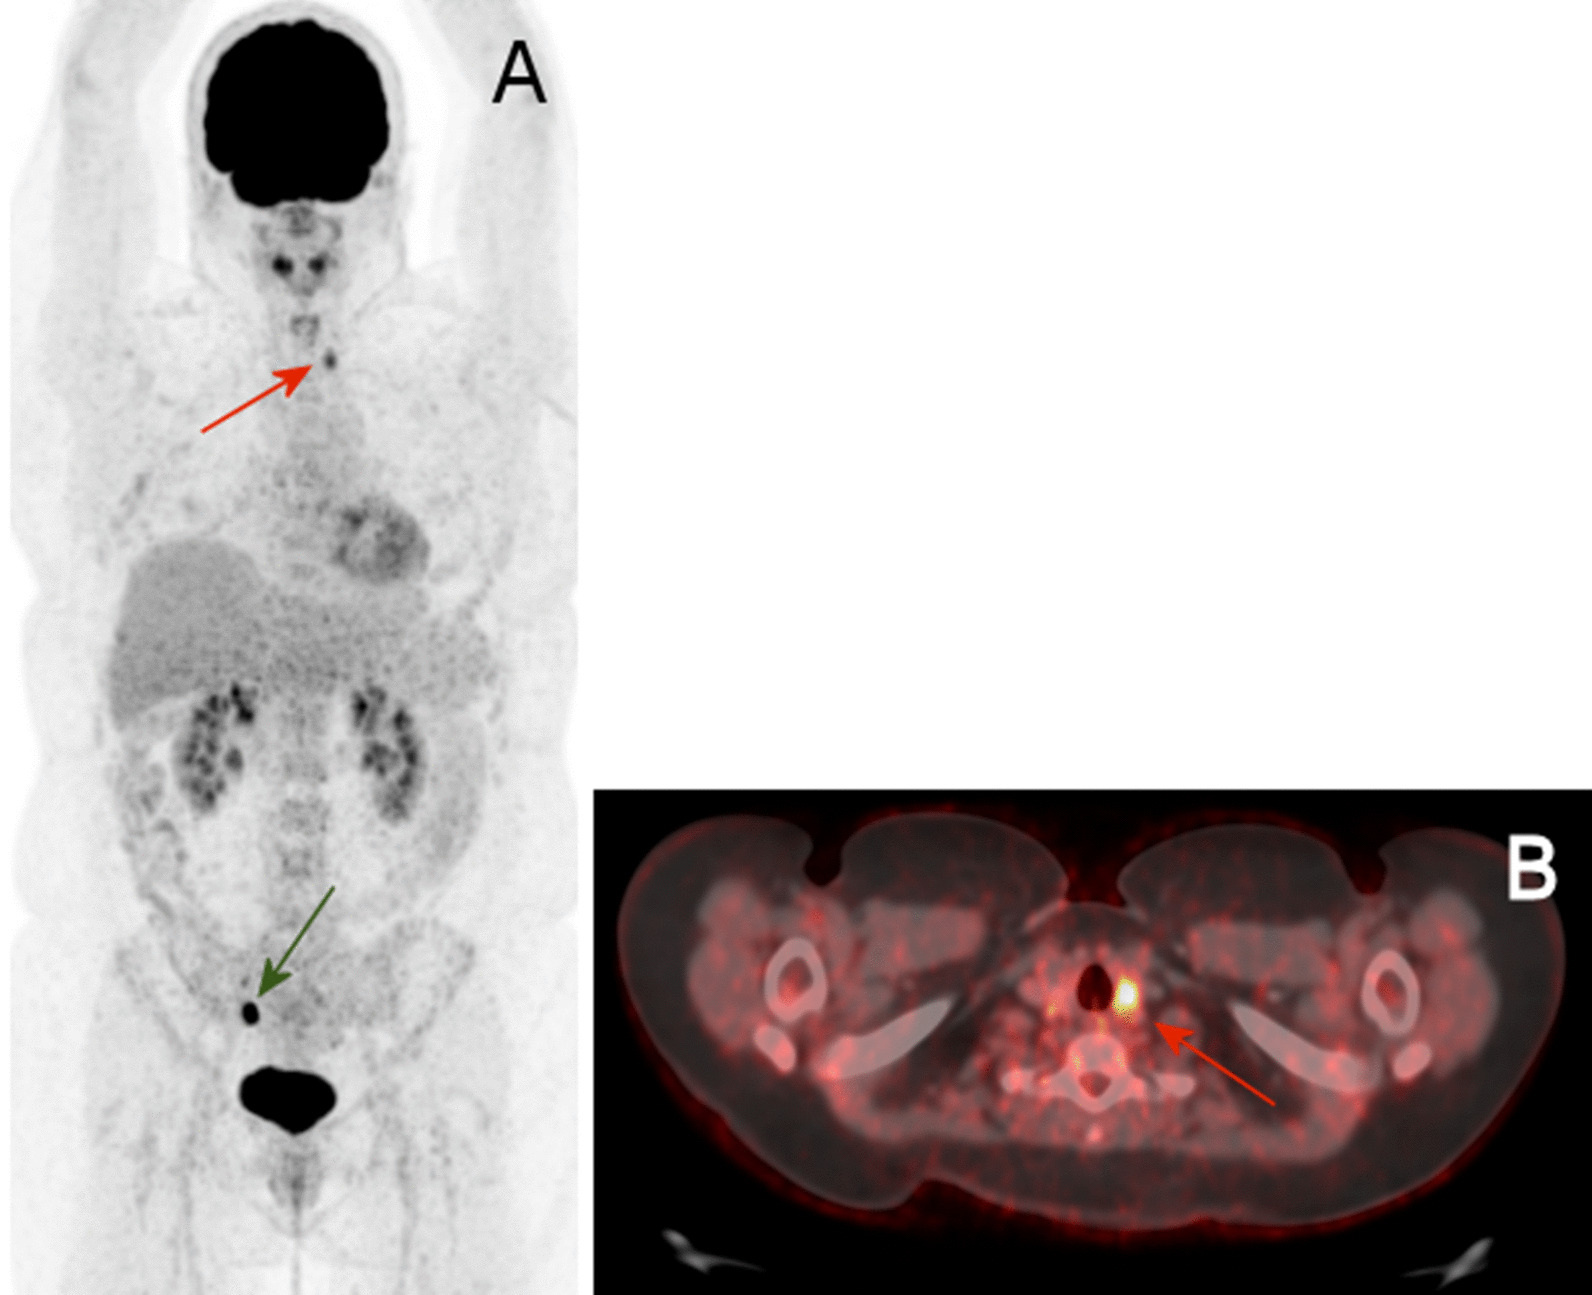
[^18^F]FDG-PET/CT in a 40-year-old woman with previous breast cancer who underwent [^18^F]FDG-PET/CT due to bone pain. [^18^F]FDG-PET/CT showed incidental focal [^18^F]FDG-uptake in the thyroid gland that revealed papillary thyroid carcinoma stage T2N1M0. A: Maximum intensity projection and B: axial section of the neck region of [^18^F]FDG-PET/CT. The red arrows show the incidental focal uptake in the left thyroid gland, and the green arrow shows incidental benign physiologic uptake in the right ovary
